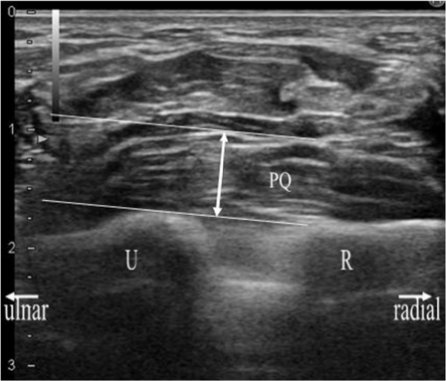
Sonographic transverse image of the examined part of the distal forearm, including anatomical reference and measurements of PQ thickness, using a 17-MHz transducer. Left hand of the same volunteer with Figure 2. We referred to the line tangential to the convex aspect of the ulna, which was drawn from the ulnar rim of the radius. PQ: pronator quadratus; R: radius; U: ulna; full-lined arrow: maximum thickness of PQ.
